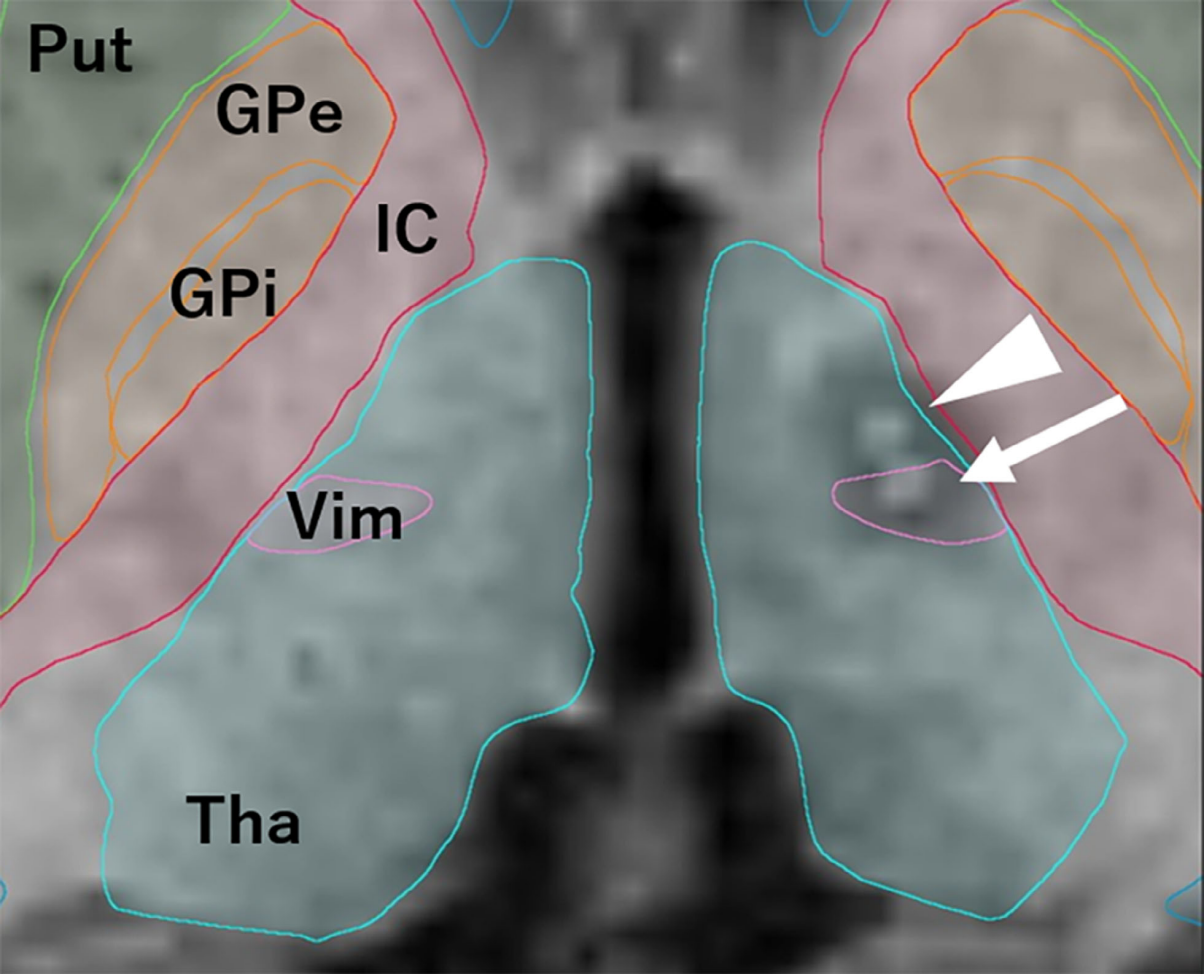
Post‐Vop‐Vim thalamotomy MRI. Radiofrequency lesions at the Vop (arrowhead) and Vim (arrow) were confirmed using the Brainlab Elements anatomical mapping tool. Thalamus (blue), Vim nucleus (pink), putamen (green), globus pallidus internus (Gpi, orange) and externus (Gpe, orange), and internal capsule (red). MRI, magnetic resonance imaging; Vim, ventral intermediate nucleus; Vop, posterior part of the ventro‐oral nucleus.
